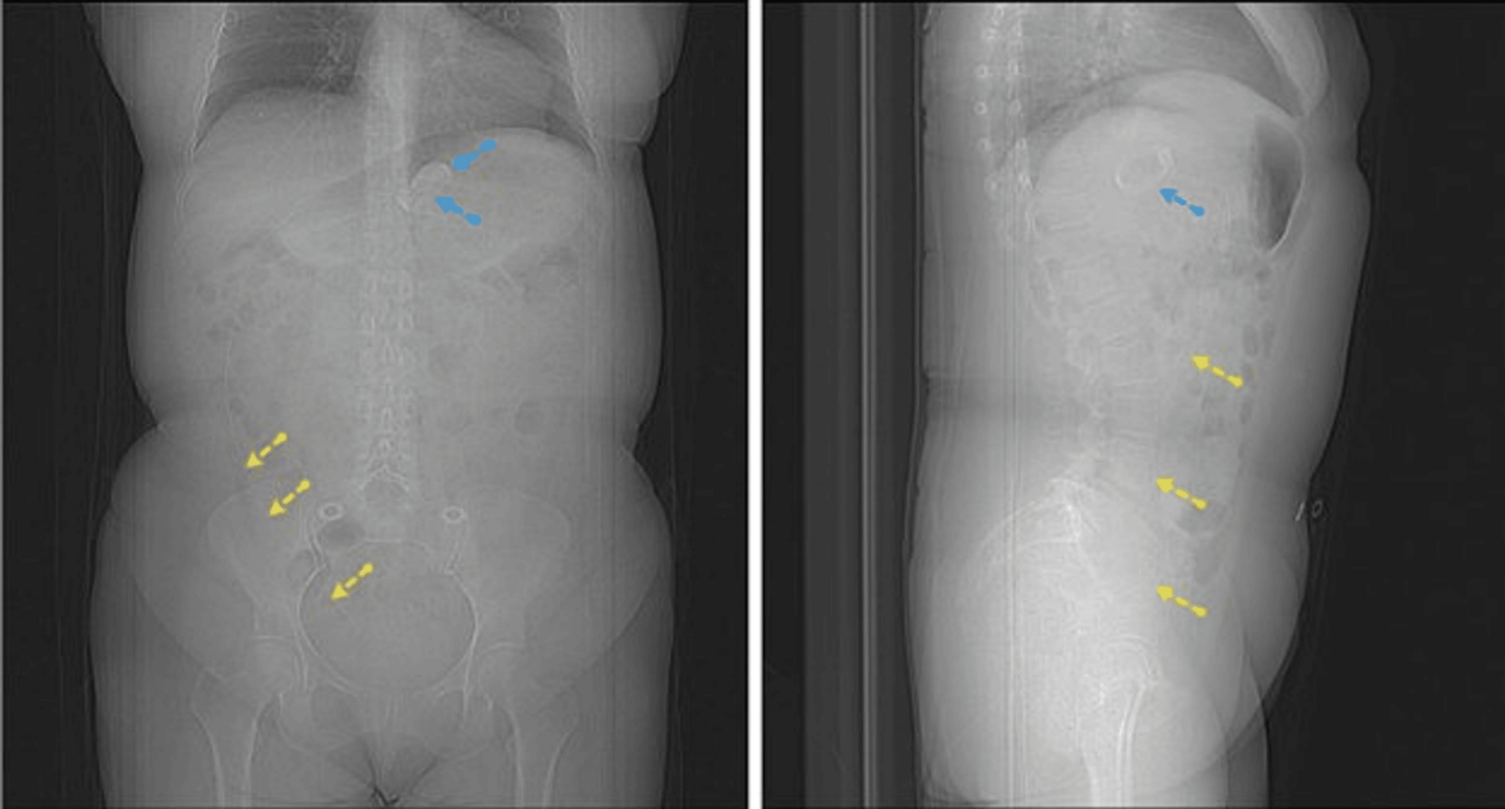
Computed tomography with contrast (left, coronal plane; right, sagittal plane). A gastric band (blue dotted arrows) and device catheter (yellow dotted arrows) were visualized. The catheter was freely suspended inside the abdominal cavity without any port attachment.
